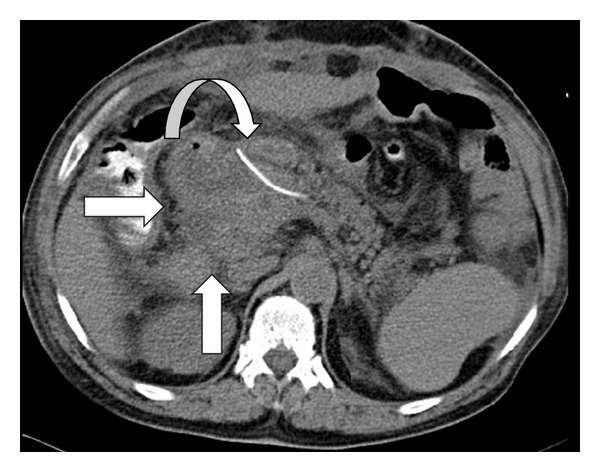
CT scan carried out on the 9th postoperative day following pancreaticoduodenectomy reveal peripancreaticojejunal anastomosis collection (straight arrows). This grade B pancreatic fistula was successfully managed by CT guided aspiration. The internal pancreatic duct stent is also seen (curved arrows).
